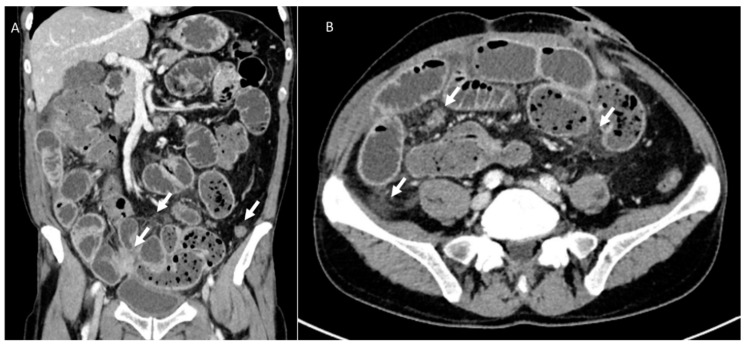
CT assessment during follow-up of rectal surgical-treated patient. In the MPR coronal plane (A) and axial plane (B), arrows show peritoneal carcinosis with intestinal occlusion.
